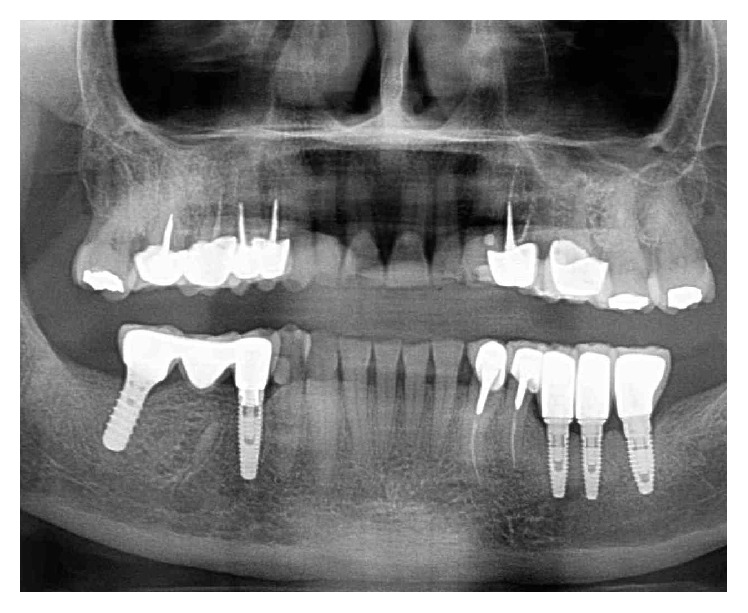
Panoramic X-ray 11 years after.
